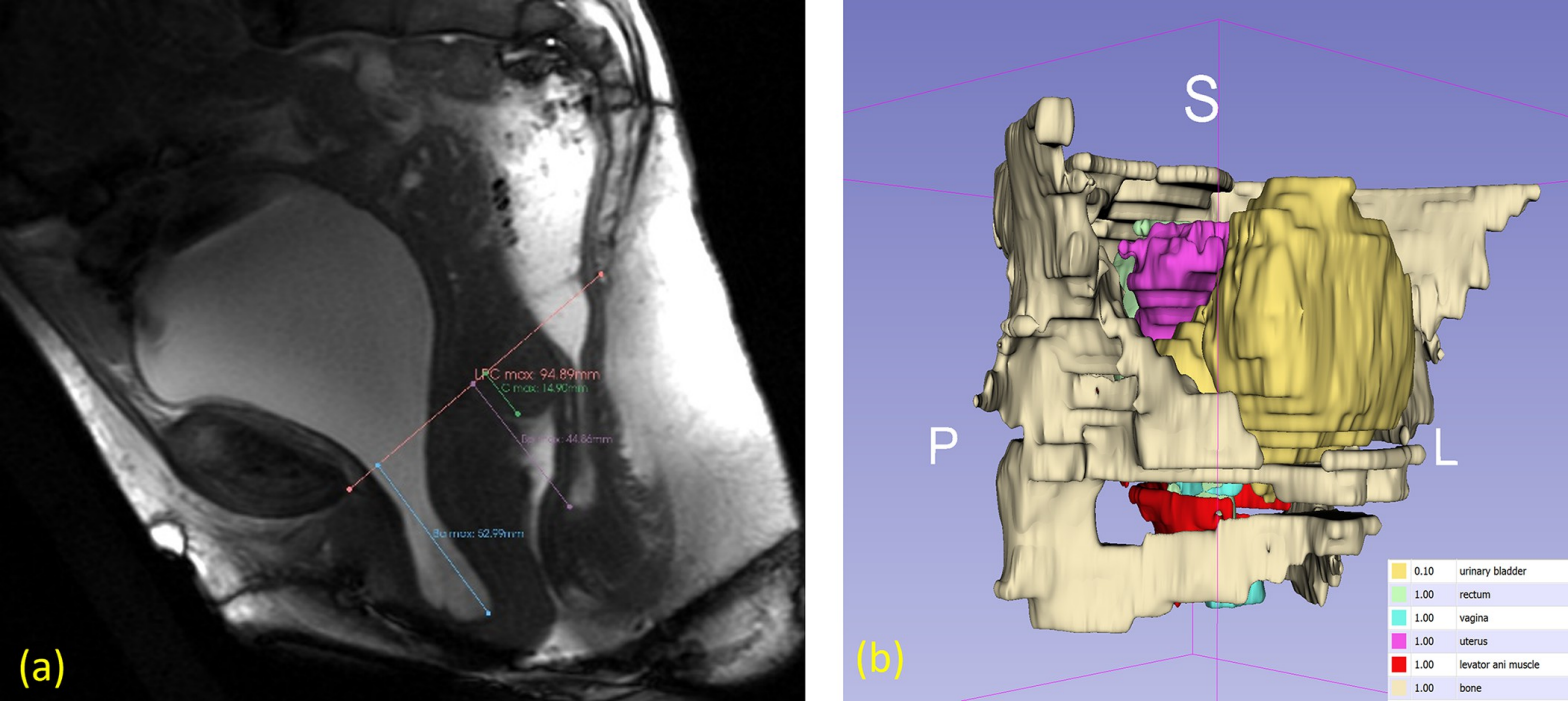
Construction of a geometric model based on the preoperative patient-specific Magnetic Resonance Imaging (MRI). (a) Pelvic organ displacement measurements (mm) on the sagittal section of the dynamic MRI under maximum strain using the pubococcygeal line (PCL) method [30]: Pubococcygeal line (pink), anterior compartment (Ba max point), cervix (C max point), posterior compartment (Bp max point). (b) 3D reconstruction of the pelvic system from the patient-specific preoperative MRI.
